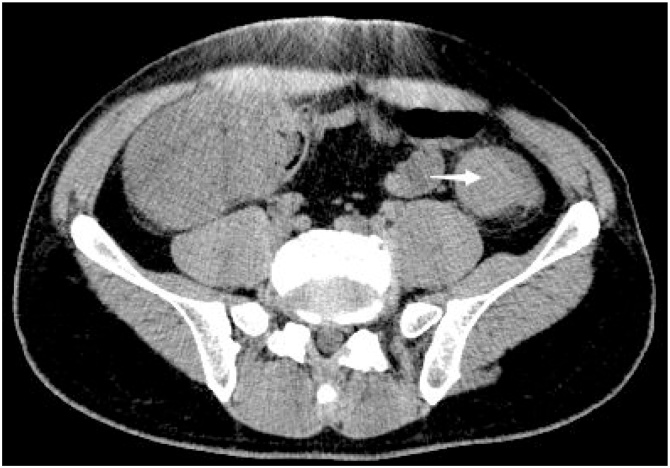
CT of abdomen and pelvis shows obstructive mass in sigmoid colon.
